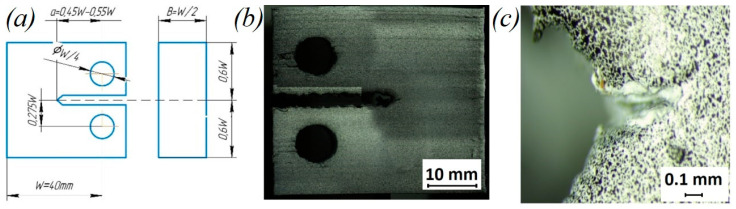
(a) Geometry of CT sample; (b) PEEK CT sample with speckles; and (c) enlarged view of crack is sharp edge area of sample.
